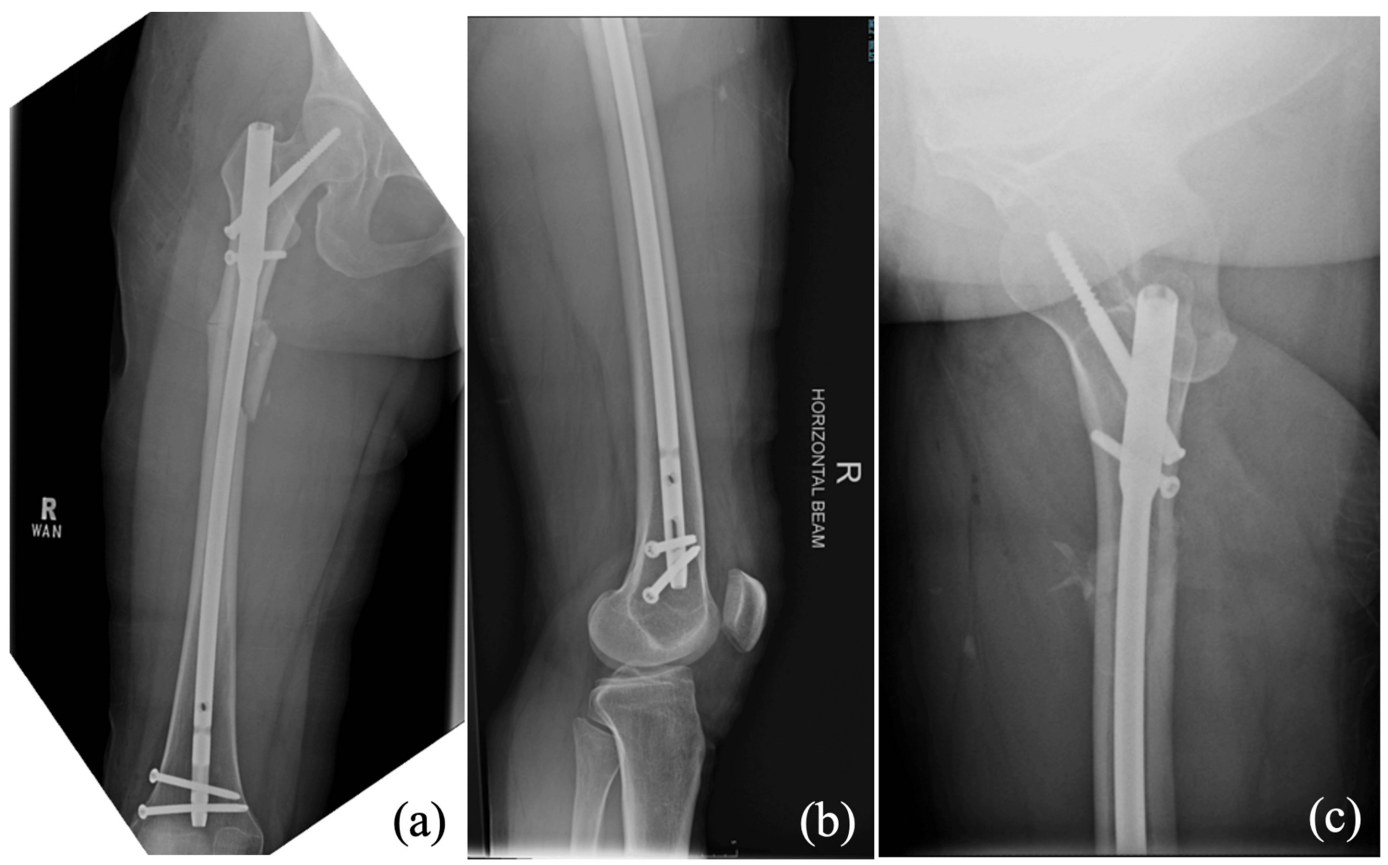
Radiographs of right femur postsurgery. (a) AP view, (b) distal lateral view, and (c) proximal lateral view
